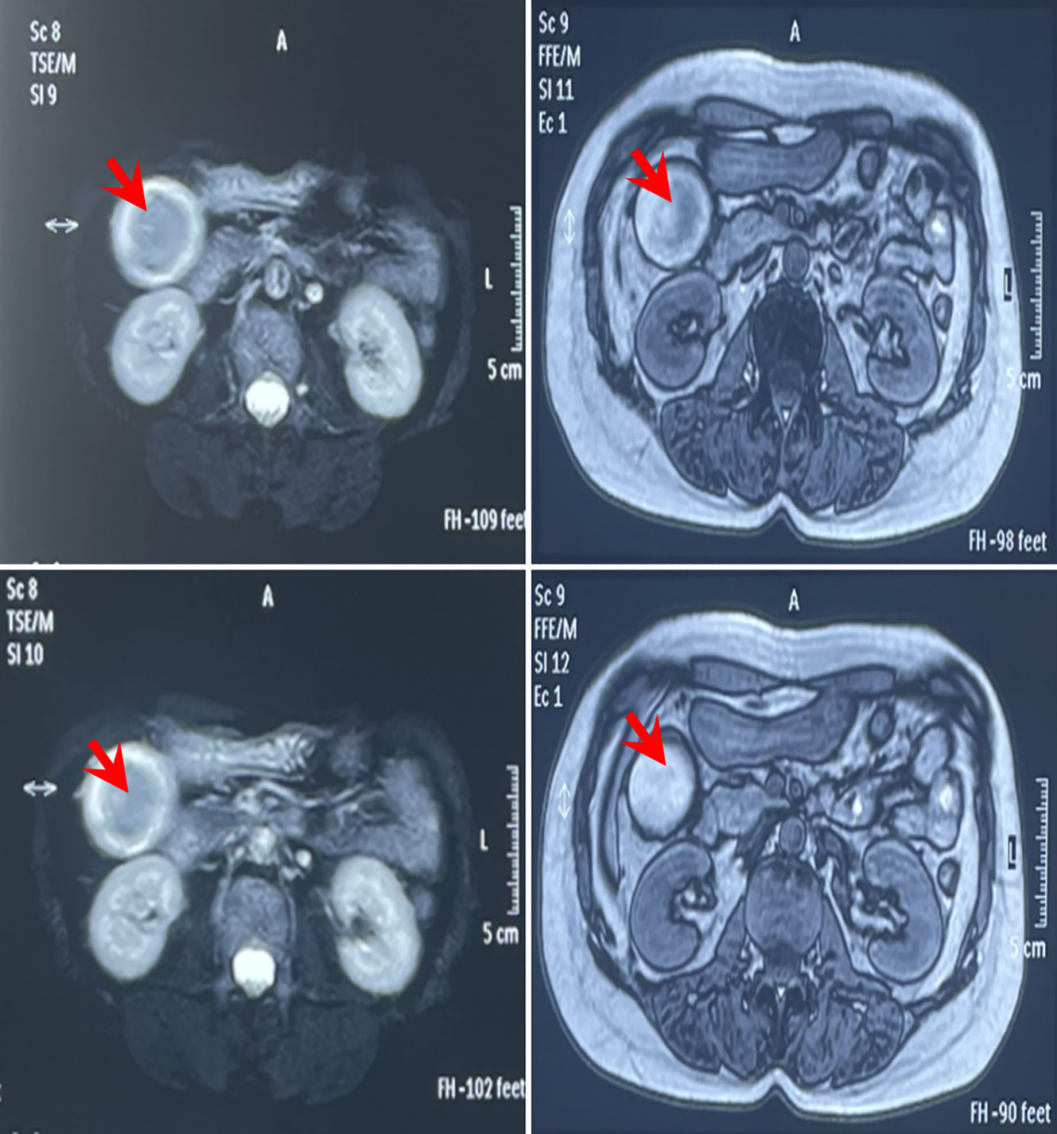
MRI imaging before operation on 9 September, 2021 of the reported case in Gaoyou People’s Hospital (Jiangsu, China). The results indicated cholecystolithiasis, cholecystitis, and cholangitis, as well as hemangioma-like changes in the left lobe of the liver (red arrow).
